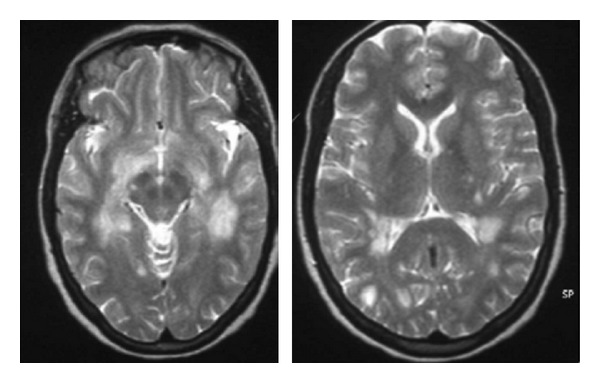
Brain MRI revealed hyperintense lesions of white matter on axial T2-weighted images.
